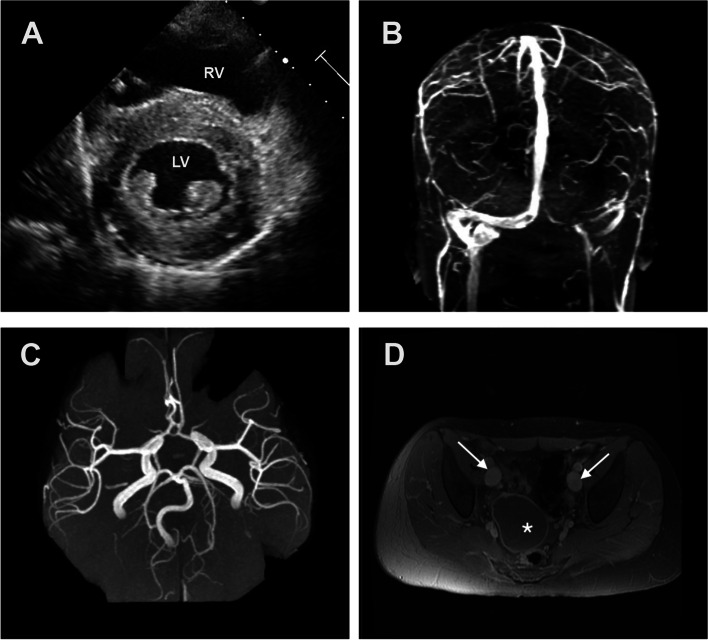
A Transthoracic enchocardiogram in the parasternal short axis view depicting left ventricular hypertrophy. B and C MRV and MRA revealing dilated and slightly tortuous dural veins and arteries of the circle of Willis. D MRI of the pelvis in the axial plane post contrast depicting dilated bilateral iliac veins (arrows) and a right adnexal cystic mass with rim enhancement (asterisk). LV: Left Ventricle, RV: Right Ventricle
